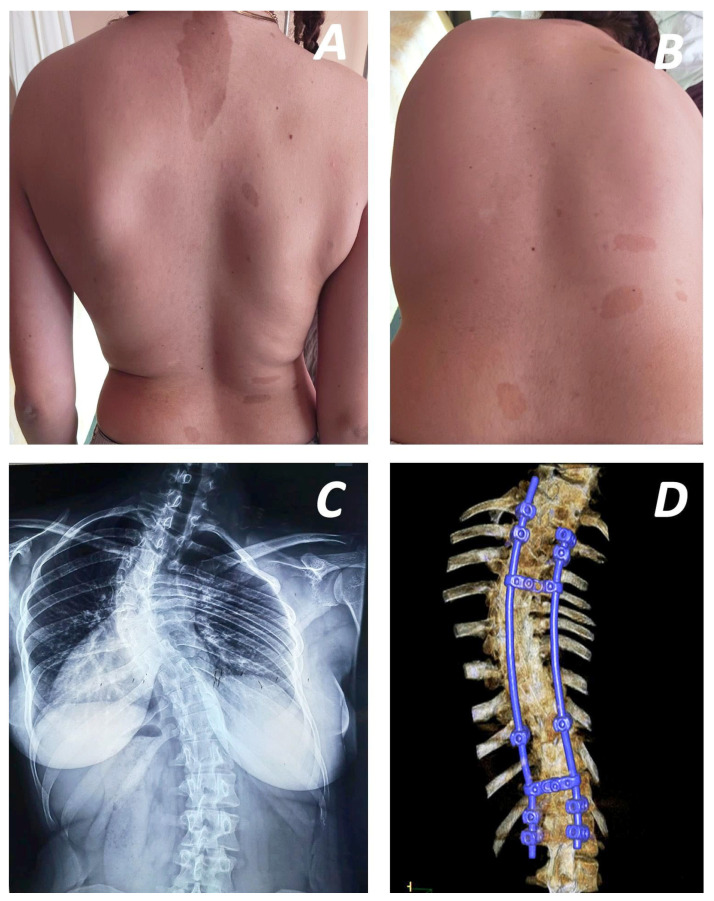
27-year-old patient suffering from dystrophic scoliotic deformity without neurological symptoms with the characteristic flat, café-au-lait spots and freckles on the skin, presenting left shoulder elevation (A) and hub at the left side of the back during Adam’s forward bend test (B). The radiographic examination revealed left thoracic scoliotic deformity of 76° between vertebrae T3 and T10 with the apex at T6 (C). Surgical correction with posterior approach and instrumentation between T1 and L2 led to partial reduction in scoliosis of 42° due to limited bony stock and hypoplastic pedicles (D).
